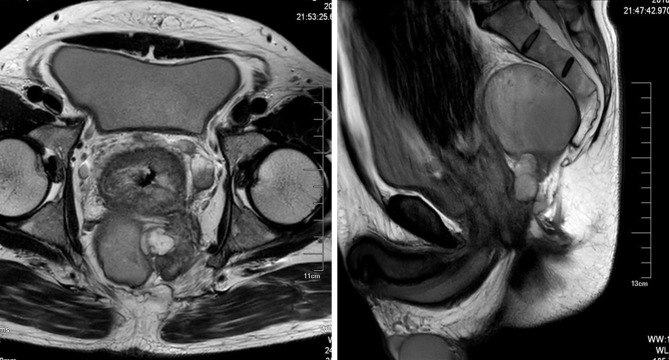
MRI: Several cysts were located in the retrorectal space, which had a clear border with the sacrum but adhered to the rectal tumor.
